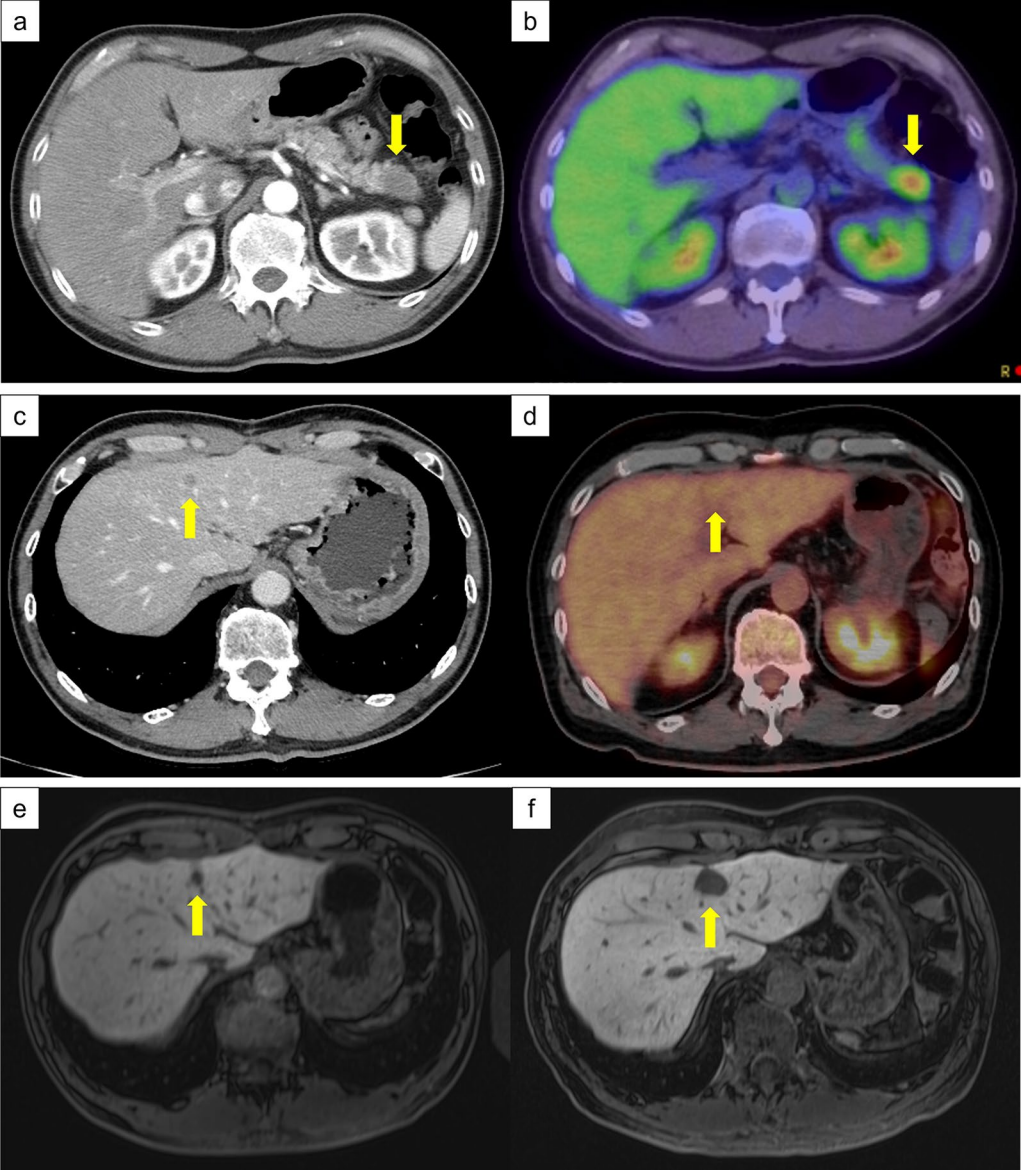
HYPERLINK "sps:id::fig1||locator::gr1||MediaObject::0" Imaging study for pancreatic cancer and liver metastasis. a Late arterial phase of dCECT revealed a hypovascular lesion at the pancreatic tail. The tumor diameter was 3.0 cm. b Positron emission tomography reveals a high standardized uptake value (maximum 6.6). c Two years after curative pancreatic resection, a small hypovascular liver lesion was identified in segment 4. The tumor diameter was 1.2 cm. d Positron emission tomography at the diagnosis of liver metastasis reveals a standardized uptake value of 3.3, which was equivalent to normal liver parenchyma. e, f Change that occurred from before to after chemotherapy for liver metastasis. Although the tumor diameter increased to 2.5 cm, the number of tumors did not change. *dCECT* dynamic contrast-enhanced computed tomography
